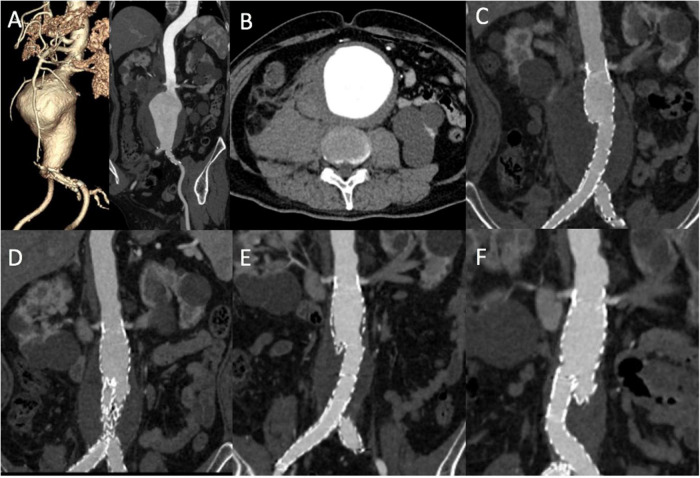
A rEVAR in a patient with a hostile proximal aneurysm neck. (A,B) Computed tomography angiography (CTA) showed a ruptured abdominal aneurysm with a short and angulated proximal neck. CTA demonstrated that the size of the aneurysm body with complete thrombosis gradually decreased after rEVAR at 3 months (C), 1 year (D), 2 years (E), and 5 years (F). rEVAR, ruptured endovascular aneurysm repair.
